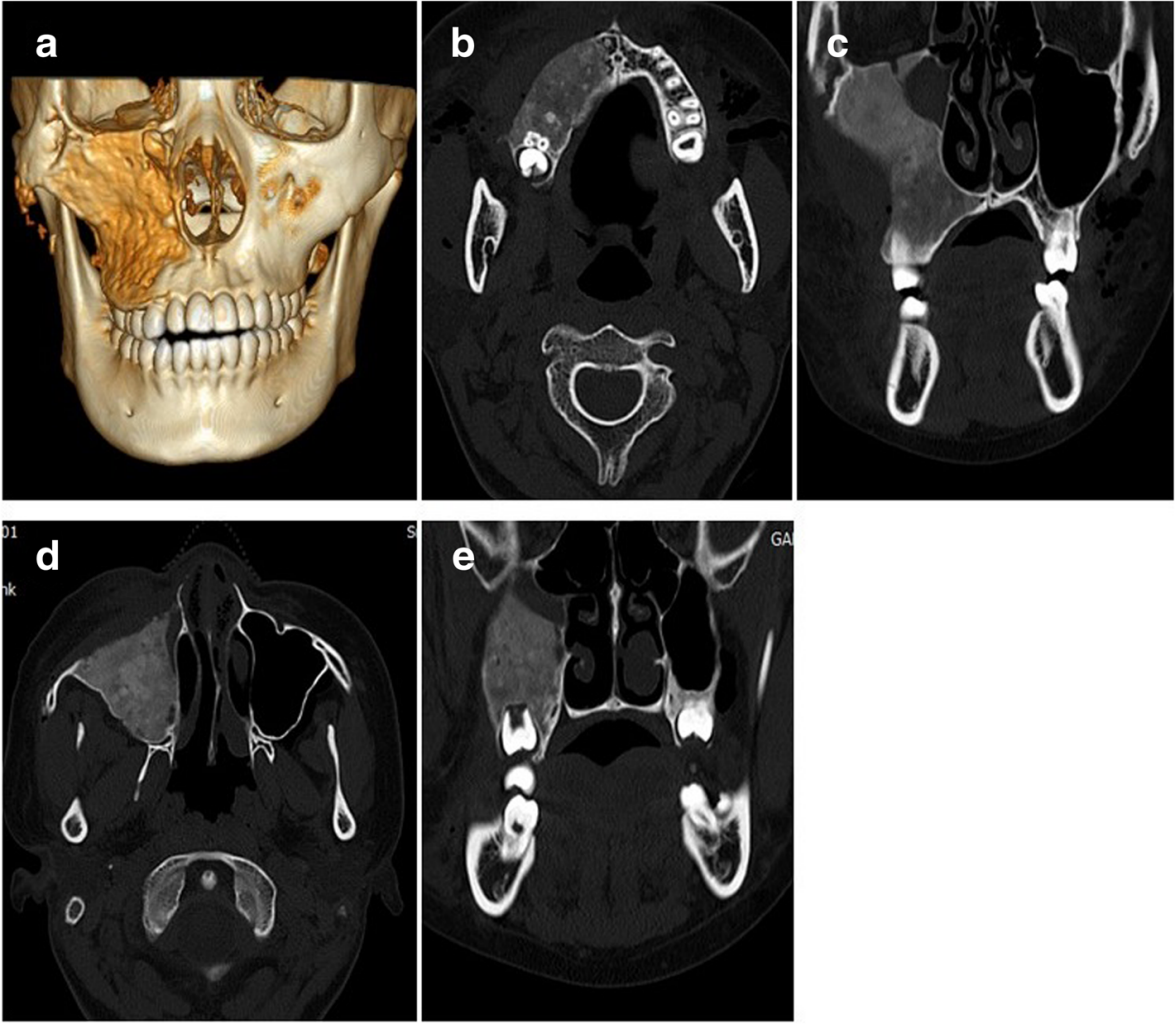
Postoperative radiographic images. a Postoperative 3D CT image showed relatively symmetrical skeletal contours and cortical bone formation on surgical site. b, d Four-month postoperative axial CT showed the restoration of the anterior protrusion of the maxilla. c, e Four-month postoperative coronal CT showed the improved contour of the right maxilla
